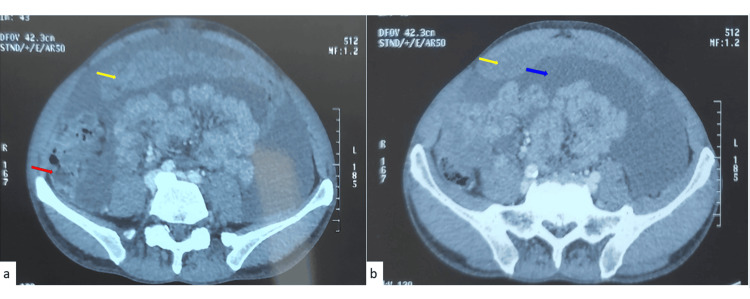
Contrast-enhanced abdominal CT scan of the abdomen and pelvis showing ileocecal wall thickening (red arrow in a), peritoneal enhancement (yellow arrow in a and b), and abundant ascites (blue arrow in b).
